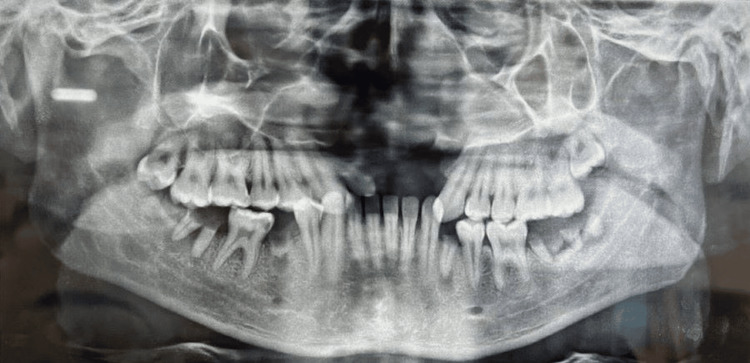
Orthopantomogram (OPG) showing missing 11, 12, 21, and 22; root stumps at 35, 37, 38, 46, and 48; supraerupted 17 and 27.
